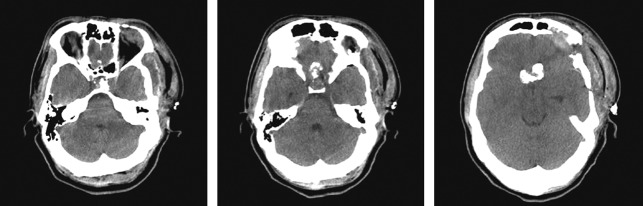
Immediate postoperative period axial CT head images showing the extent of surgical resection with residual calcified tumour.
